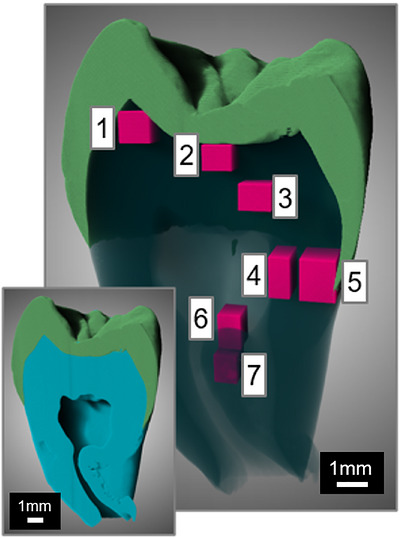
Example sample locations used in this study (inset: Computed tomography scan of Tooth #1 used for representation). Locations are denoted as follows: (1) under cusp, (2) under fissure, (3) bulk coronal, (4) transitional pulp, (5) transitional periphery, (6) upper root, (7) lower root.
